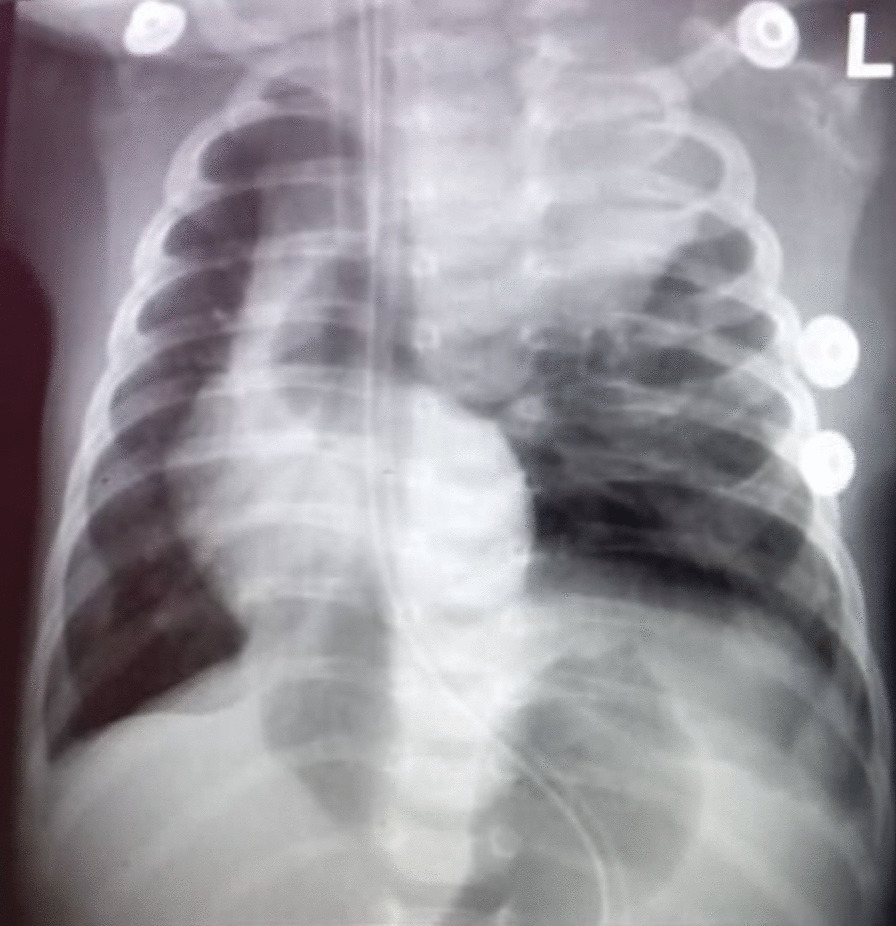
Chest X-ray done a few hours post-surgery, showing right mediastinal shift and left upper lobe of the lung with hypoplasia
